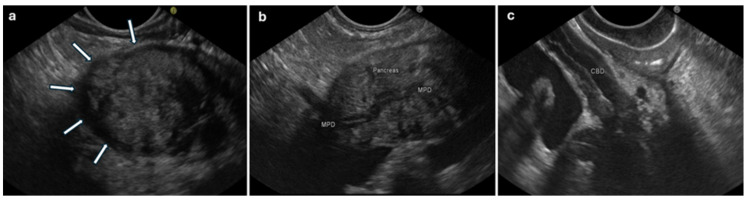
Endoscopic ultrasonography in autoimmune pancreatitis. (a) Hypoechoic rim around pancreatic parenchyma (arrows). (b) Visible unobstructed main pancreatic duct (MPD) with thickened wall: “duct-penetrating sign”. (c) Thickened wall of common bile duct (CBD) with 3-layer-type appearance.
